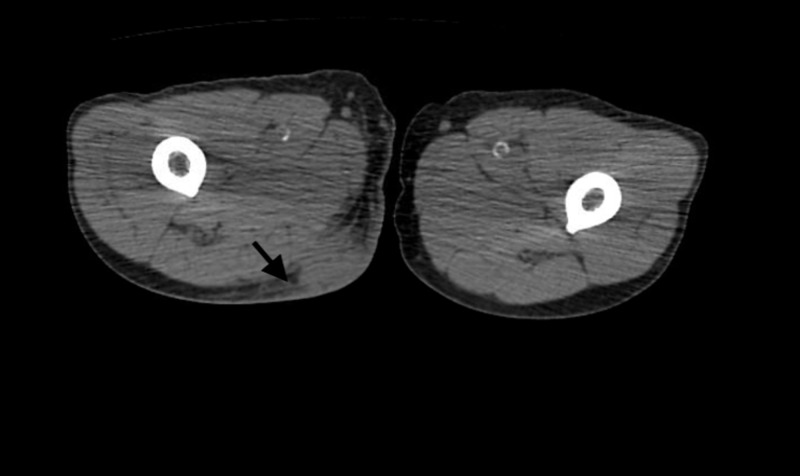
CT pelvis and bilateral lower extremities without contrast showed a 3.5-cm fluid collection (arrow) in the right posterior thigh
